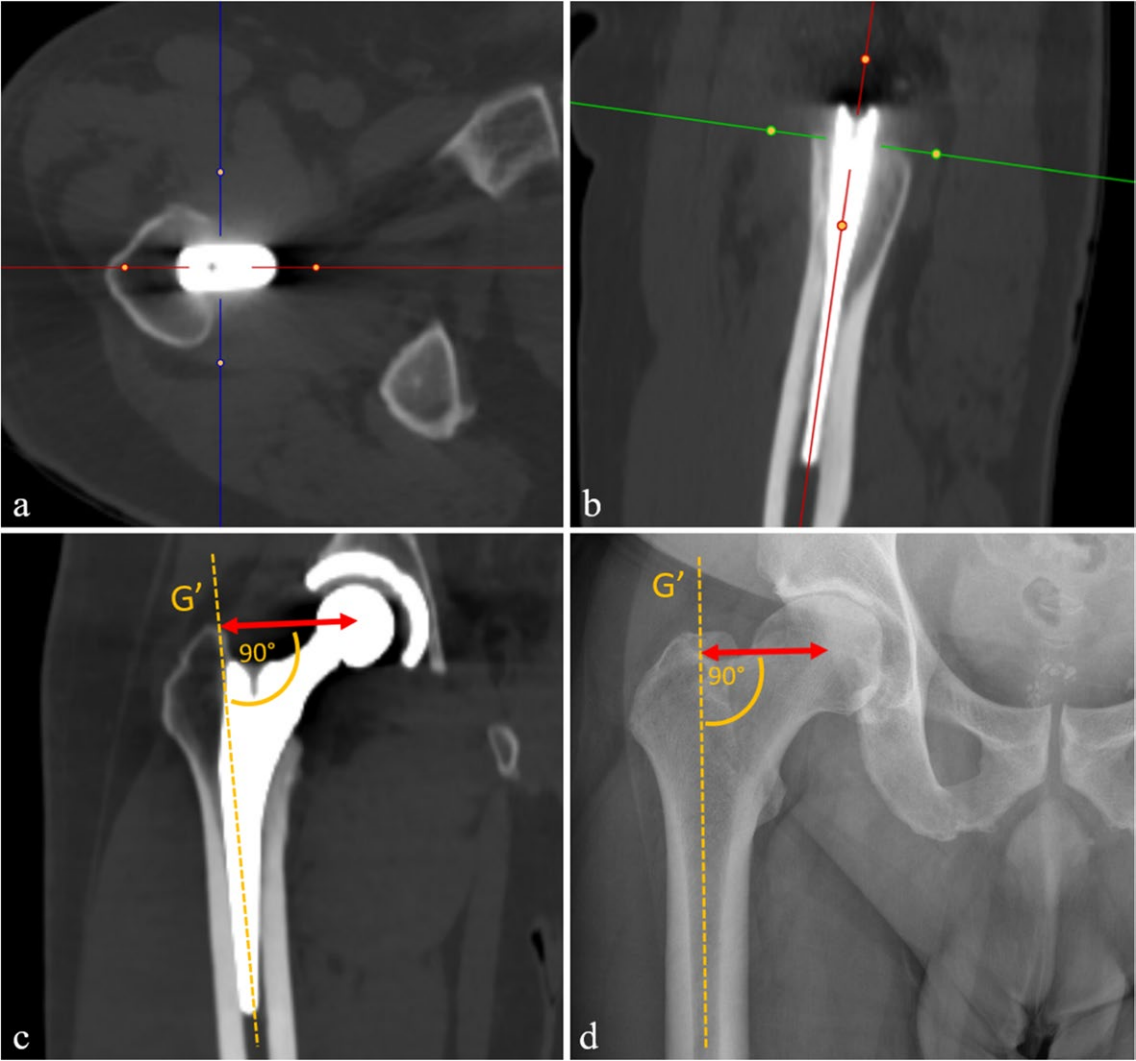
For calculation of femoral offset on CT images, these were adjusted utilizing multiplanar reformation along the axes of the prosthesis shaft in the axial and sagittal plane (a, b). Subsequently, a line was drawn along the femoral shaft (*G*′), intersecting the most prominent point of the greater trochanter. Femoral offset (double-arrow) was then calculated as the distance between the femoral head center of rotation and a line bisecting the femoral long axis (c). The same method for calculation of the femoral offset (double-arrow) was applied for pre-surgical radiographs of the pelvis (d)
